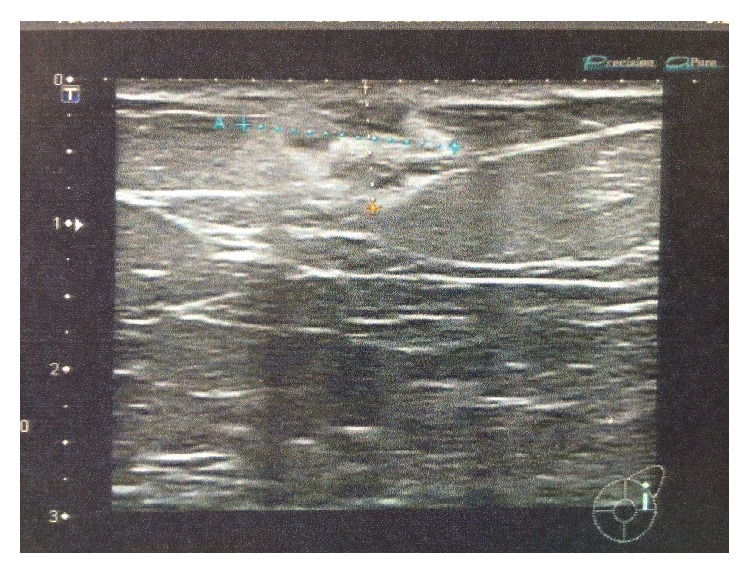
Ultrasound: heterogeneous oval mass with echogenic rim; BI-RADS ACR4.
